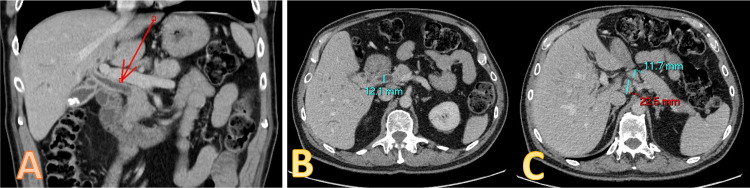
Abdominal contrast-enhanced computed tomography. (A and B) Diffuse common bile duct thickening and biliary dilatation. (C) Few peri-pancreatic slightly enlarged nodes.
